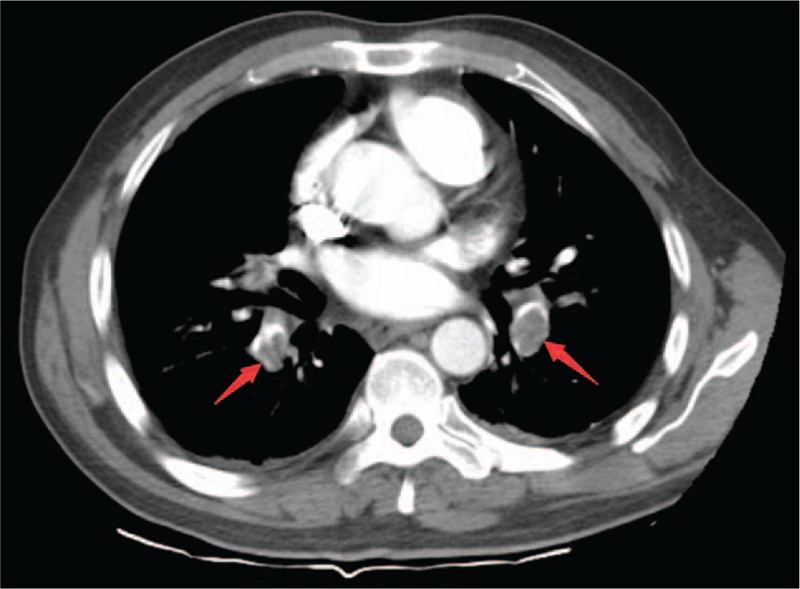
CTPA revealed multiple embolism in bilateral pulmonary artery (arrow). CTPA = computed tomography pulmonary angiography.
